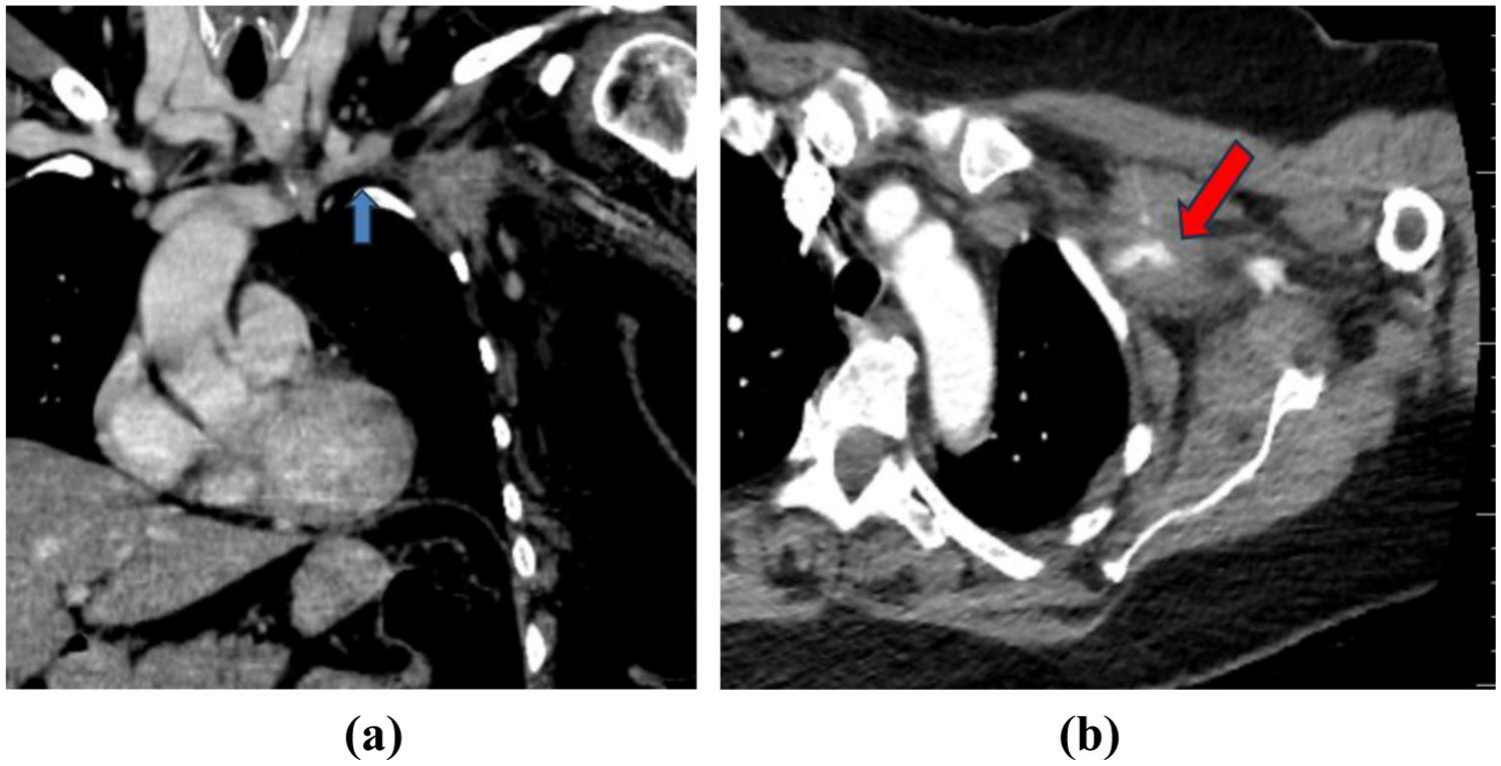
(a) Chest CT coronal section with IV contrast—venous time—arrow indicates thrombus in the left subclavian vein, in continuity with the tumor (invasion/tumor thrombus). (b) Chest CT axial section with IV contrast—arterial time –arrow indicates the embedded subclavian/axillary artery, still permeable.
